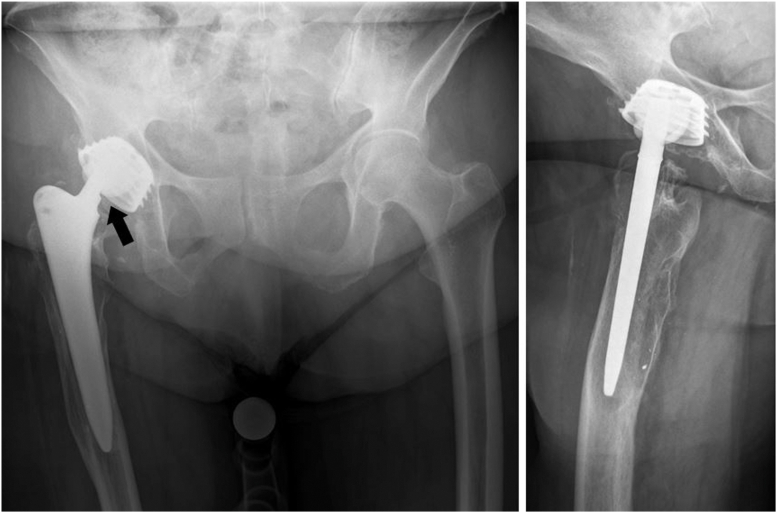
Preoperative plain radiographs, anteroposterior, and right axial pelvis radiographs taken 4 years prior to the patient presented at our institution. Findings indicative of ceramic liner fracture include the head’s asymmetric position in relation to the cup and the Femoral Neck-Bite Sign signifying damage to the stem due to abrasions (black arrow). Large osteolytic areas are present already.
